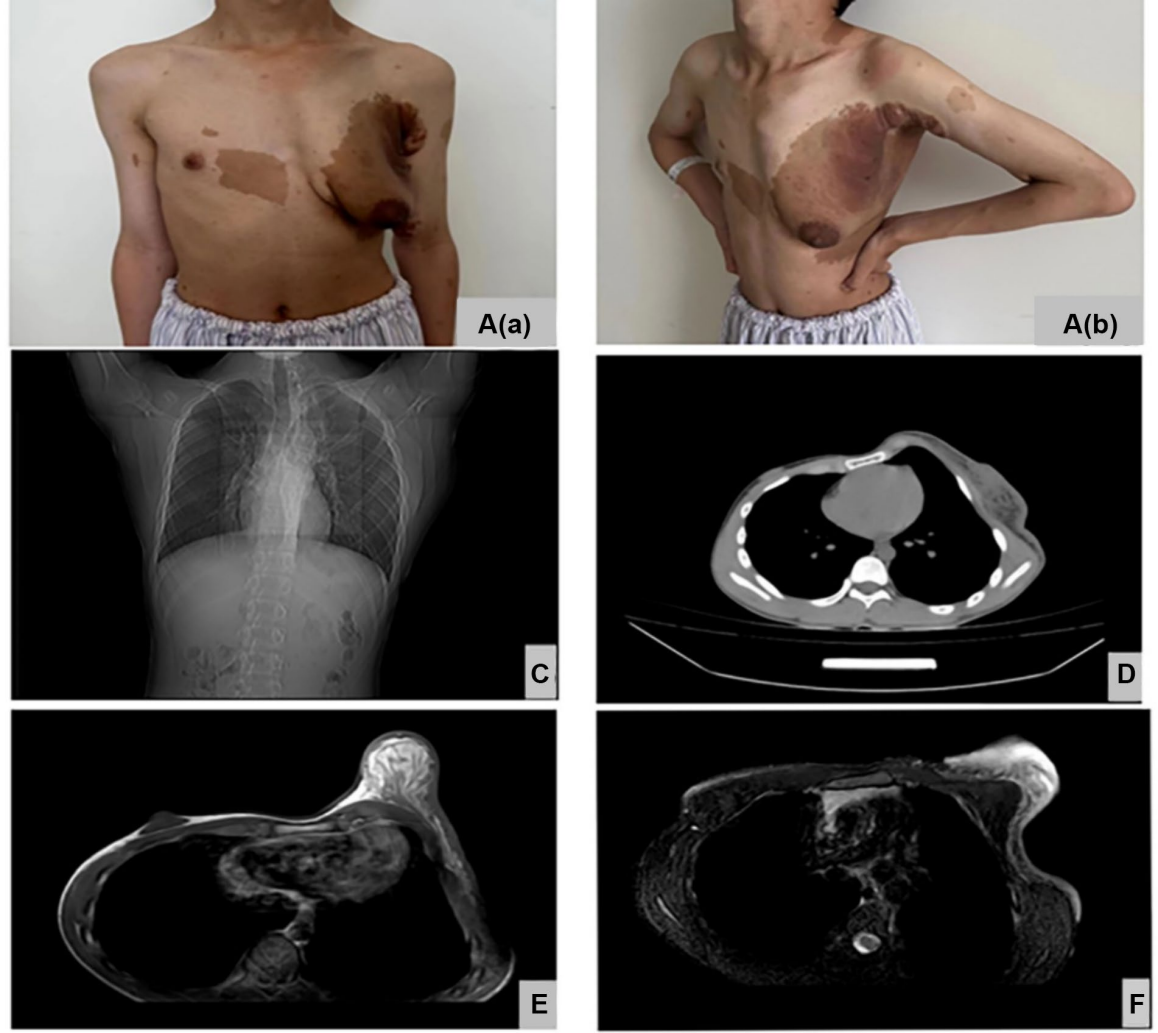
Shown the front view of patient (A(a)) Lateral view of patient (A(b)), CT showing thoracic scoliosis and scoliosis (B), CT showing increased glands in the left breast (C), Breast MRI showing male breast development (D), Breast MRI showing edema in the left breast, skin and anterior chest wall (E).
